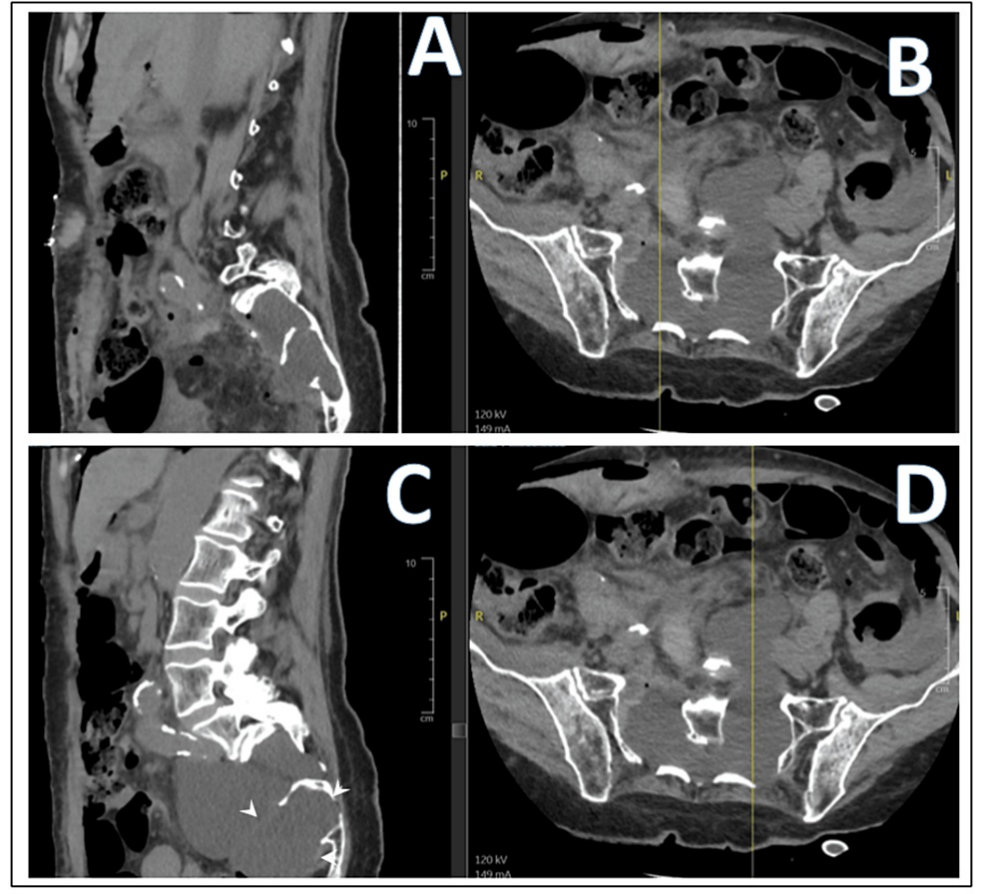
Postoperative abdominal CT. Sagittal (A) and axial (B) images focusing on the right upper sacral meningocele, and sagittal (C) and axial (D) images focusing on the left lower meningocele.
